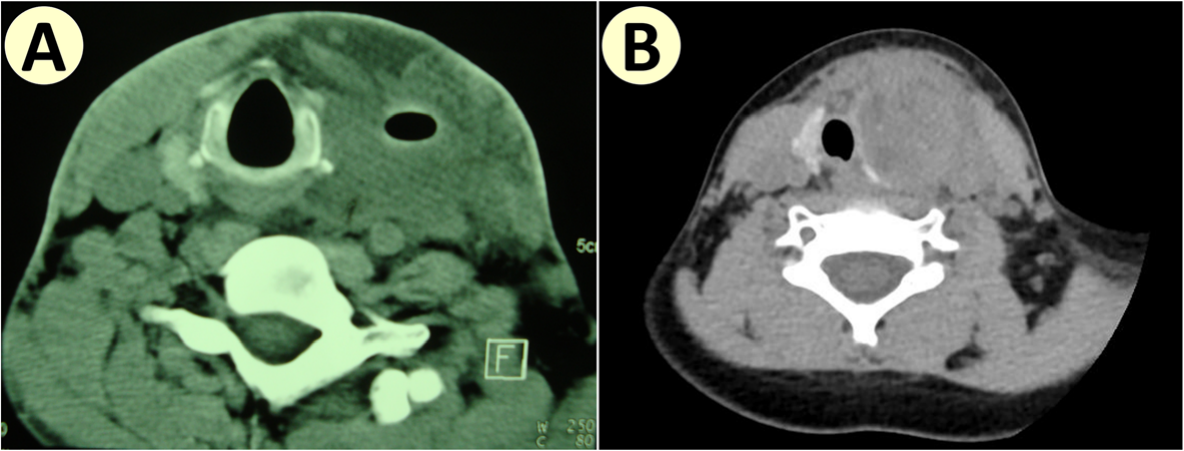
Close relationship between fourth branchial fistula and thyroid gland on CT scan. a Left-sided infected fourth branchial fistula, whose tract can be seen at the center of the inflamed mass. b Left-sided infected fistula, whose tract isn’t obvious
